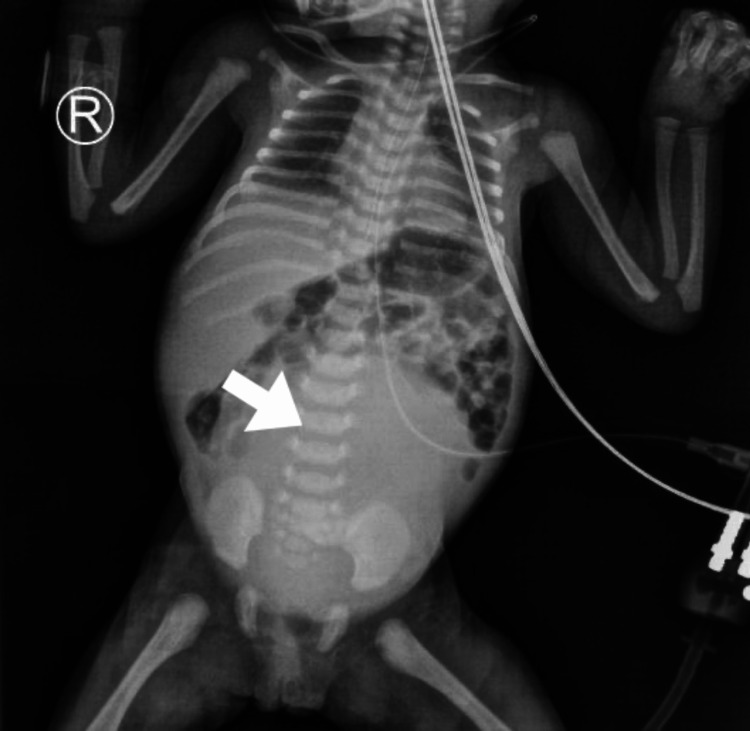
X-ray showing a large pelviabdominal cystic lesion shadow at the abdomen with no pathological calcifications.
